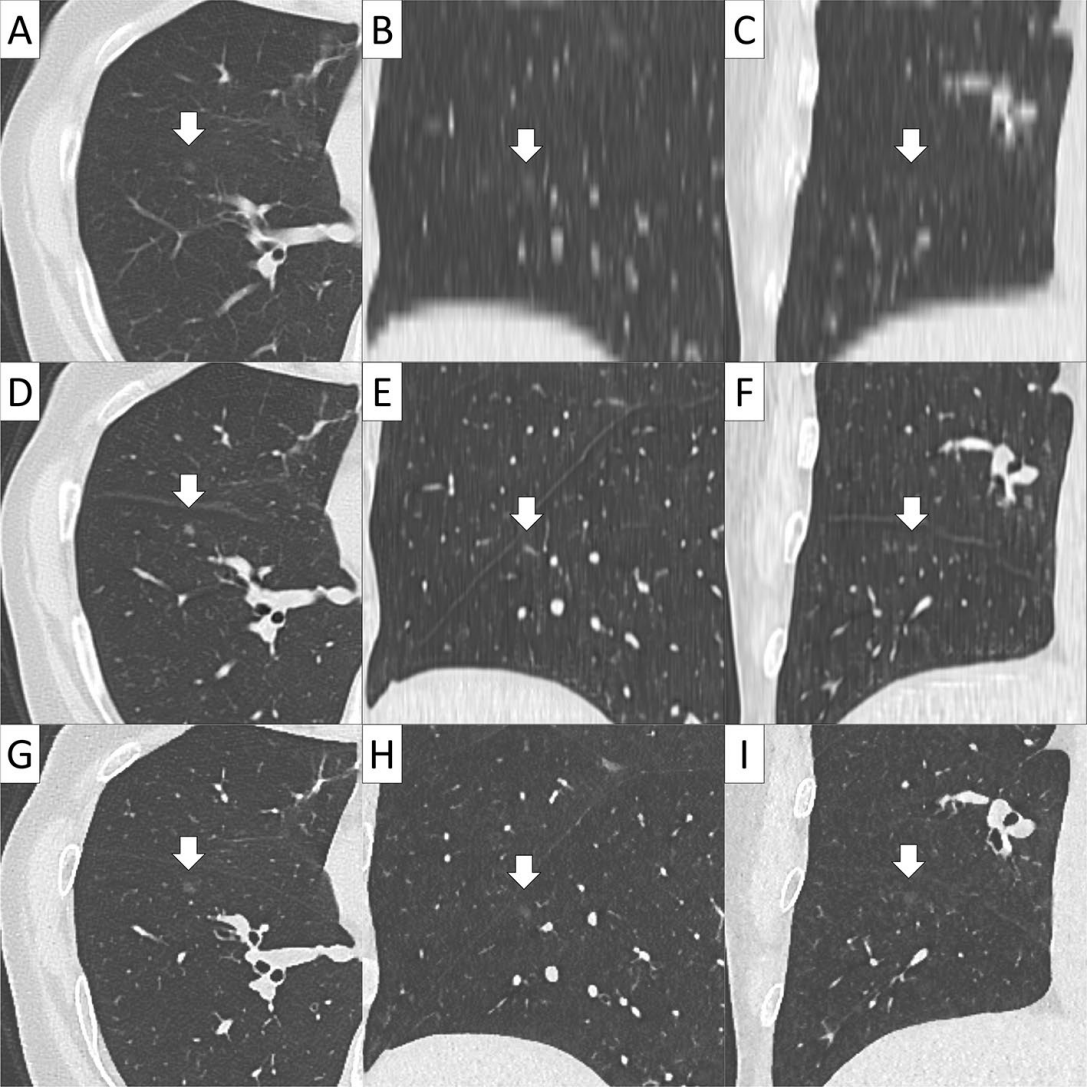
Adenocarcinoma in situ with a diameter of 5 mm in a 60-year-old female. (A) Axial view, (B) sagittal view, and (C) coronal view of conventional 5-mm thick CT. (D) Axial view, (E) sagittal view, and (F) coronal view of virtual thin-section CT (TSCT). (G) Axial view, (H) sagittal view, and (I) coronal view of real TSCT.
